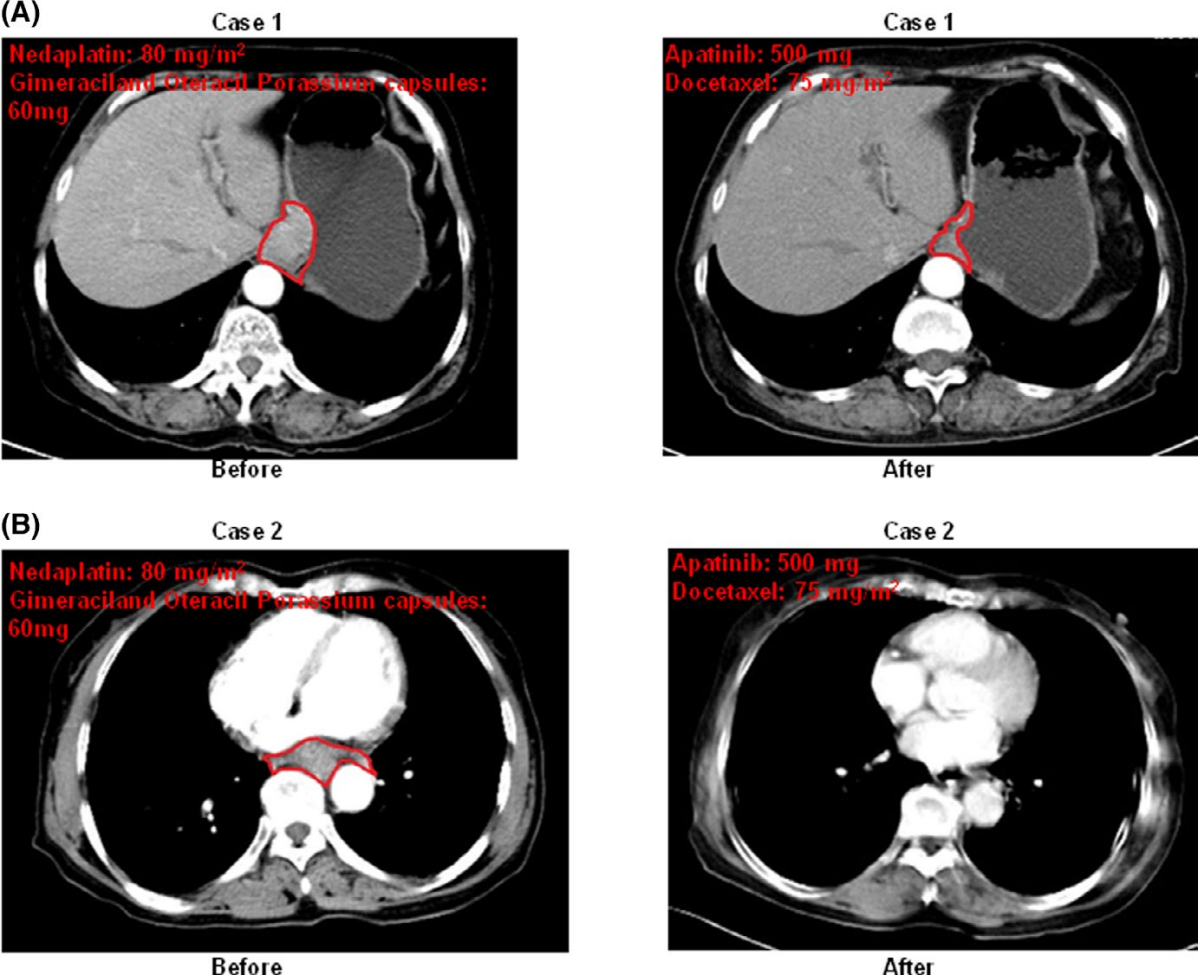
Computed tomography (CT) was used to detect the lesions before and after treatment with combination of apatinib and chemotherapeutic drug. The range of lesions were marked with red dotted lines (R, right; L, left). Case 1(A) was diagnosed with ESCC and retroperitoneal lymph node metastasis; case 2(B) was diagnosed with ESCC and mediastinal lymph node metastasis. Patients received treatment of Nedaplatin and Gimeraciland Oteracil Porassium Capsules. Nedaplatin 80 mg/m^2^ (intravenously guttae, ivgtt) was given on Day 1; Gimeraciland Oteracil Porassium capsules were given 60 mg (P.O.) twice a day in first 14 days. When the new lesions occurred, they were treated with the combination of apatinib and docetaxel. Apatinib was administered 500 mg (P.O.) once a day continuously; docetaxel 75 mg/m^2^ (ivgtt) was given on Day 1
